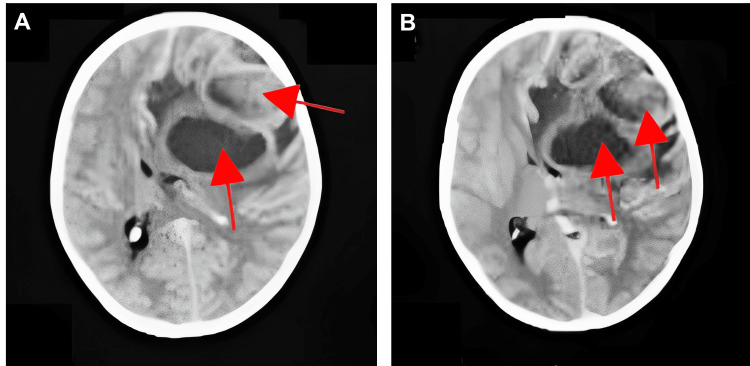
Representative slides (A-B) of cranial computed tomography revealing hypodense masses in the temporal and frontal lobes compatible with brain abscesses. Red arrows show three well-defined hypodense masses (17-20 Hounsfield units) in the frontal-parietal region with hyperdense halos with an average length of 5.3 mm and surrounding edema, which displaces the midline.
